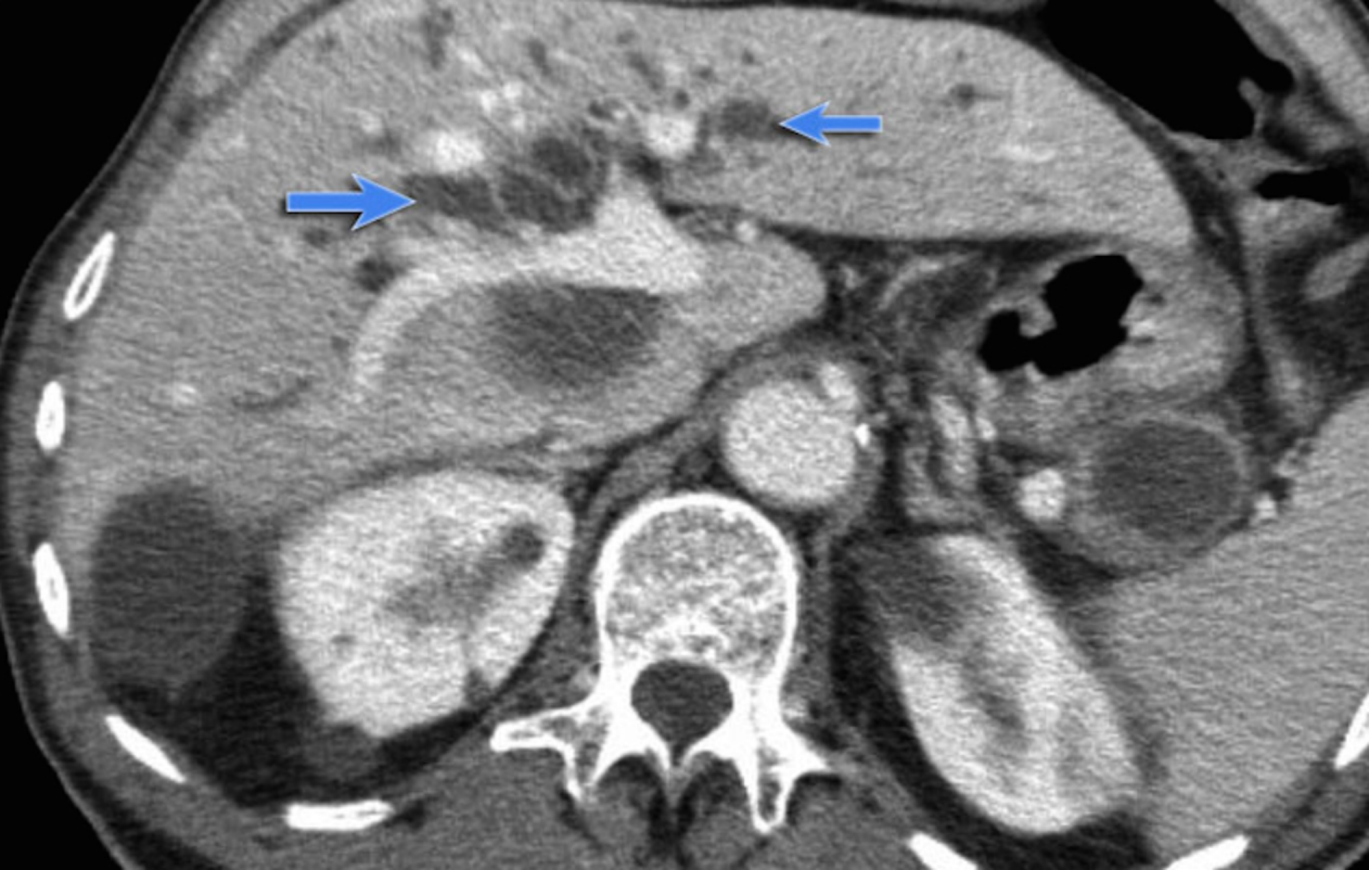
CT scan of the abdomen showing a main-duct IPMN indicated by arrows [5]; CT: computed tomography, IPMN: intraductal papillary mucinous neoplasm
